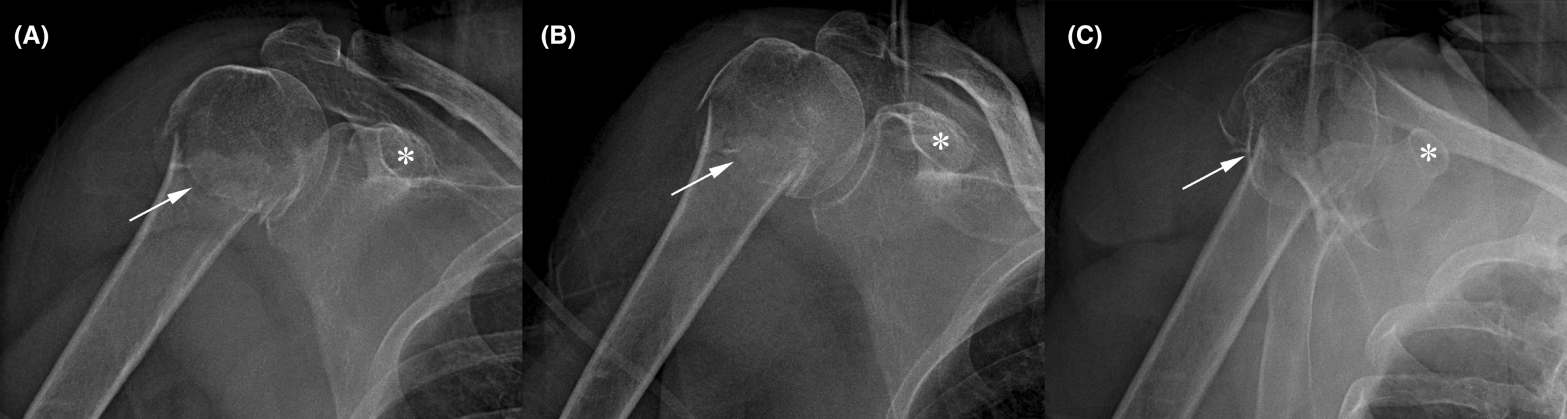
Radiographs showing our patient's minimally displaced and mildly comminuted two‐part surgical neck humerus fracture in anterior–posterior (A, B) and lateral (C) views. Asterisks (*) indicate the coracoid process and arrows indicate the fracture. Mild lucency along the fracture site seems consistent with fracture of poor‐quality bone, but some readers might consider this as subtle lysis to be consistent with a subacute infection.
